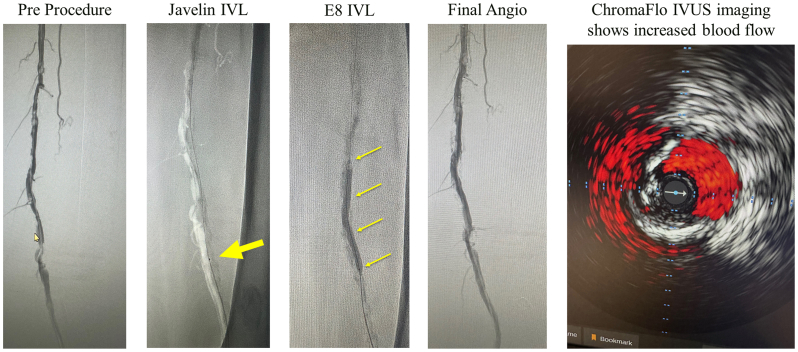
In this case of a multilevel superficial femoral artery (SFA) lesion, the full 120 pulses of the Javelin intravascular lithotripsy (*IVL*) catheter were concentrated in the most calcified regions, and an E8 IVL catheter was used to treat the remaining length of the lesion. *IVUS*, Intravascular ultrasound.
